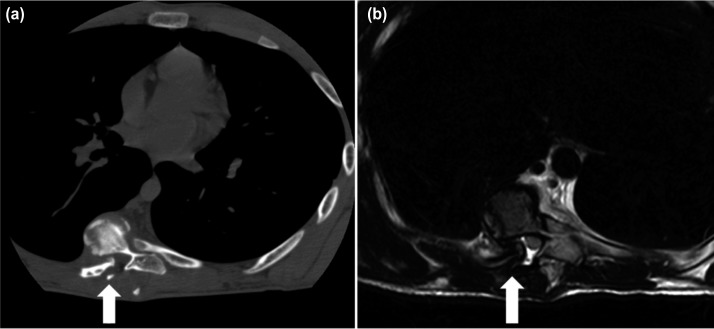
Axial cut imaging showing a dislocated right-sided T8 rib head into the spinal canal; (a) CT scan, and (b) T2-weighed MRI where the dura is indented but with no obvious cord compression (see arrows).
